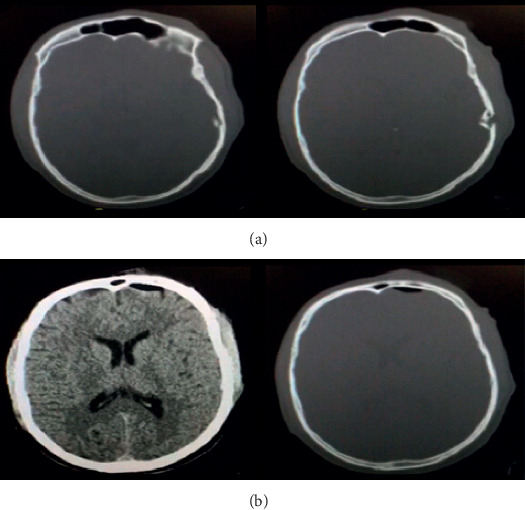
(a) CT of the brain, bone window demonstrating a left temporal depressed fracture with intra-axial bone fragments; (b) CT of the brain demonstrating a left frontal pneumocephalus in both brain window and bone window along with left temporal subgaleal swelling.
